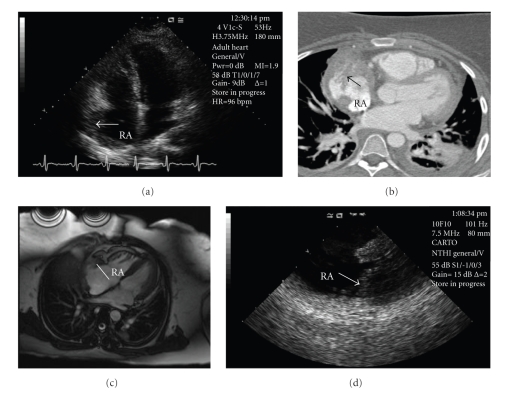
Right atrial mass imaging by different modalities: (a) Transthoracic echocardiography, apical four-chamber view. Thickening of the right atrial wall (arrow). (b) Chest CT, axial view. Enhancing mass (arrow) with papillary projections in the lateral wall of the right atrium. (c) Cardiac MRI, axial view. A heterogeneous, vascular mass involving the right atrial lateral wall. (d) Intracardiac echocardiography. Irregular mass (arrow) infiltrating the right atrial wall.
